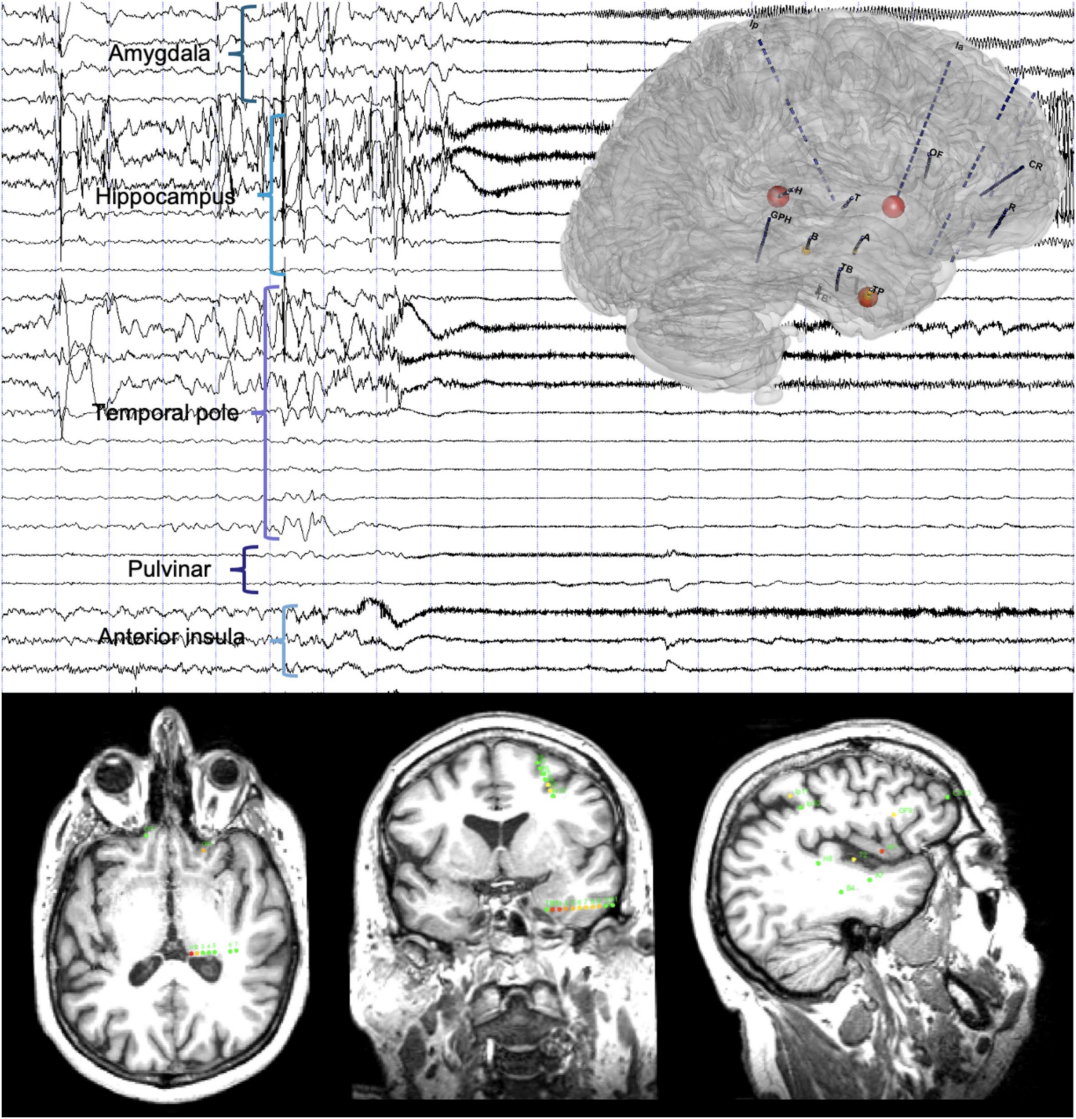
Timing of the thalamic implication in a temporal lobe seizure. Top: A 20-s SEEG recording dataset including a temporal lobe seizure and three-dimensional representation of the epileptogenicity index (EI). Seizure-onset is characterized by a DC-shift with low-voltage fast activity involving the anterior insula and the temporal pole, rapidly involving the pulvinar, and later amygdala and hippocampus. Bottom: Axial (left), coronal (middle) and sagittal (right) slices of T1-weighted brain MRI with a representation of the normalized EI values on the respective intracranial contacts (for details on the EI, see Bartolomei et al. (31), involved contacts represented according to a color scale from yellow to red, green contacts non involved in the discharge): Ia1-2 (anterior insula, right), EI = 1; H1-2 (pulvinar, left), EI = 0.97; TP2-3, TP3-4 and TP4-5 (temporal pole, middle), EI = 0.89 to 0.44.
